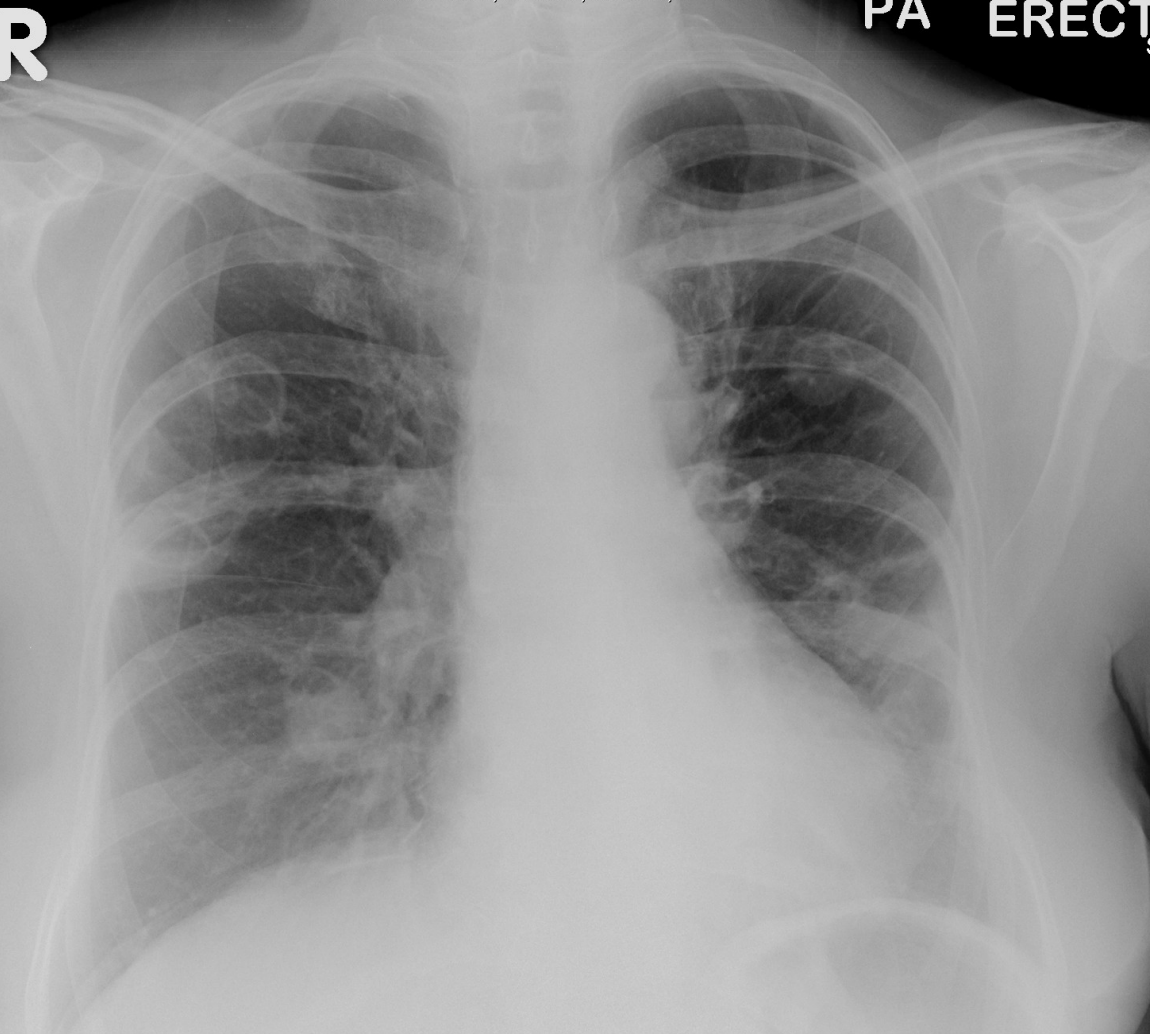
X-ray Chest – Cavitating pneumonia.
